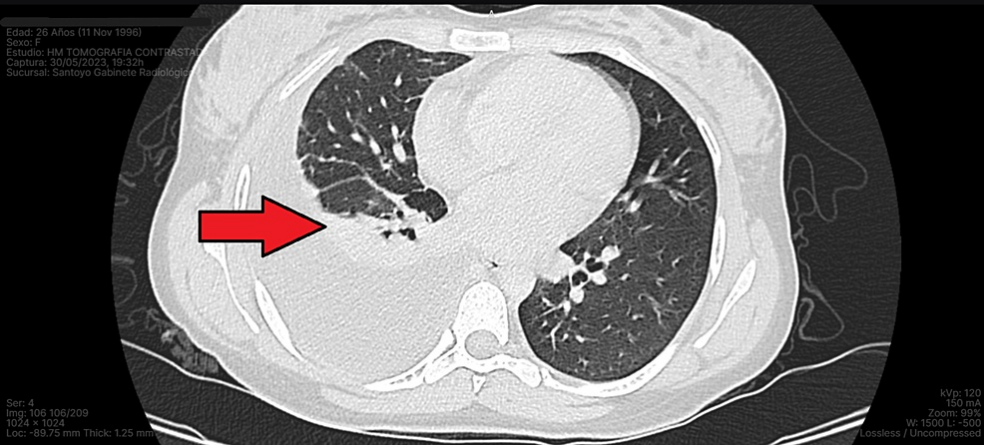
CT scan of the chest lung window showing right-sided pleural effusion
